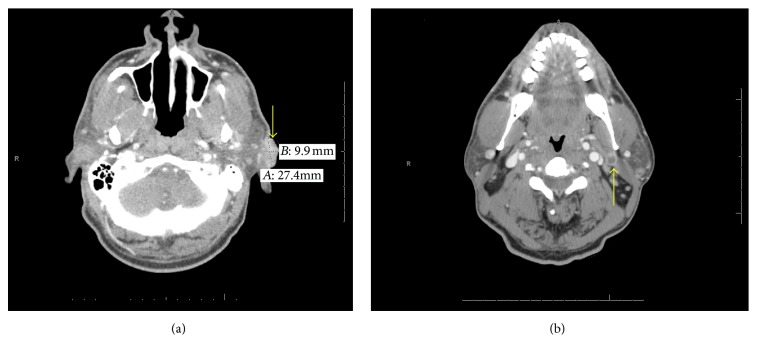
CT scan demonstrating primary tumor within the left cheek (a) and a necrotic lymph node (b).
